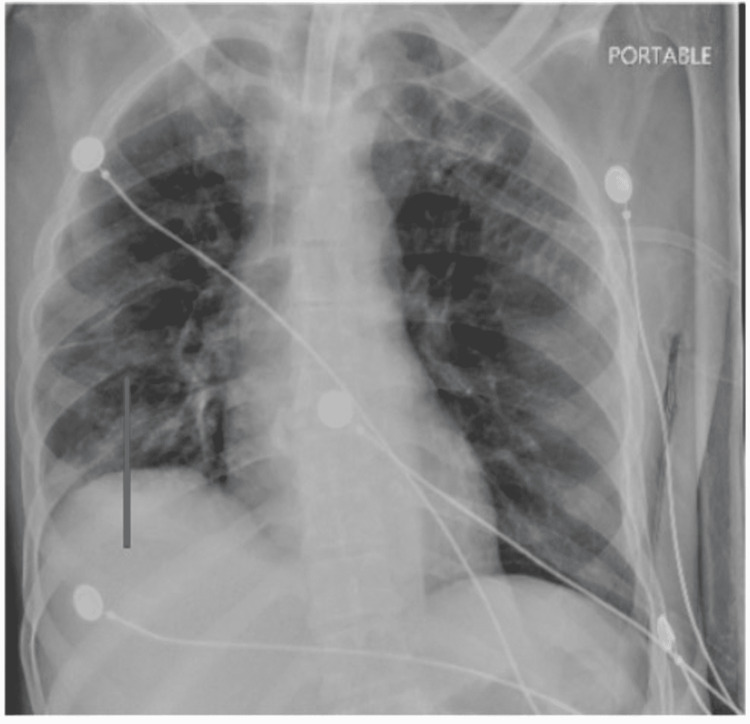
Chest X-ray showing patchy bilateral airspace disease, greatest in the right mid to lower lung. Taken during current patient admission.
